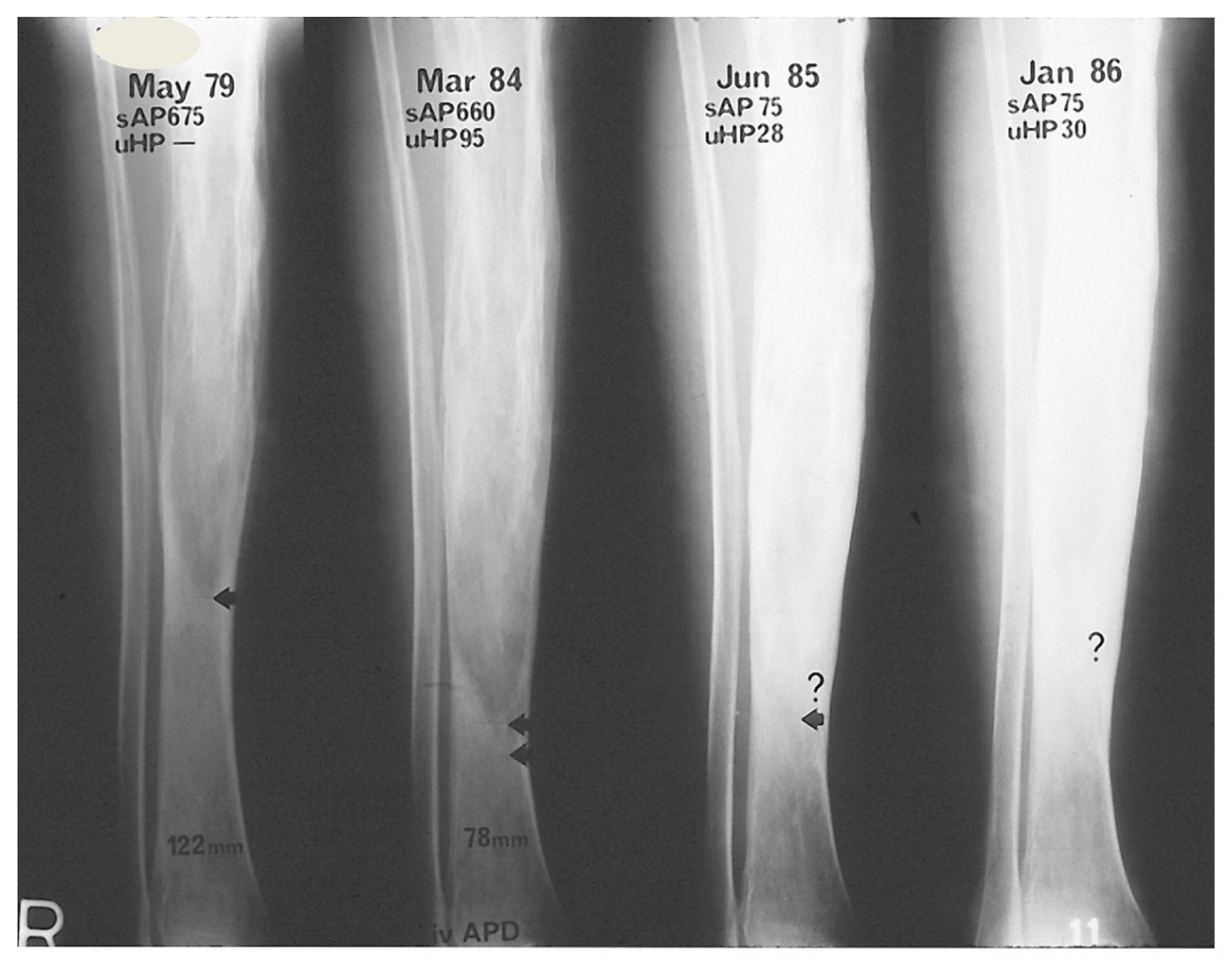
Healing of lytic bone disease in Paget’s disease of the tibia with bisphosphonate. The dates of radiographs are shown, together with the serum alkaline phosphatase (sAP) activities and urine hydroxyproline (uHP) excretions at that time. Between the first two images, no treatment was given, and the lytic wedge (indicated by arrows) progressed. At the time of the second radiograph, a course of pamidronate infusions was administered. One and two years later, the lytic lesion was healed and there was no further progression of pagetic changes along the tibia. iv APD, intravenous 3-amino-1-hydroxypropylidene-1,1-bisphosphonate, or pamidronate. The late H.K. Ibbertson provided this image to the author Ian Reid with permission to use it for publication purposes. © IR Reid.
